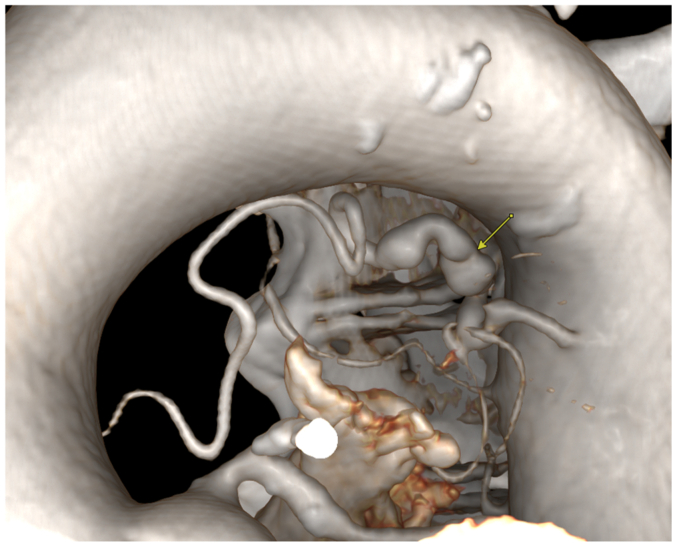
3 D reconstructed CTA images of the bronchial artery small aneurysms resembling string of beads, *yellow arrow*.
